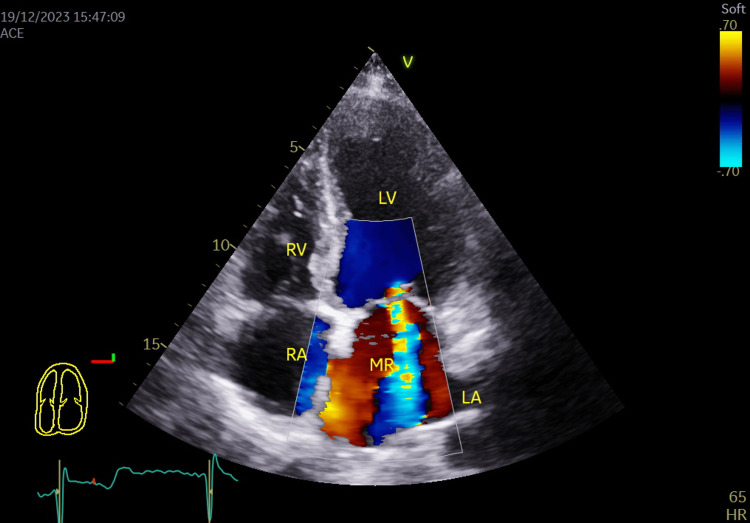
Color flow coding showing grade III mitral regurgitation in apical 4 chambers view. LA: Left atrium; LV: Left ventricle; RA: Right atrium; RV: Right ventricle; MR: Mitral regurgitation
